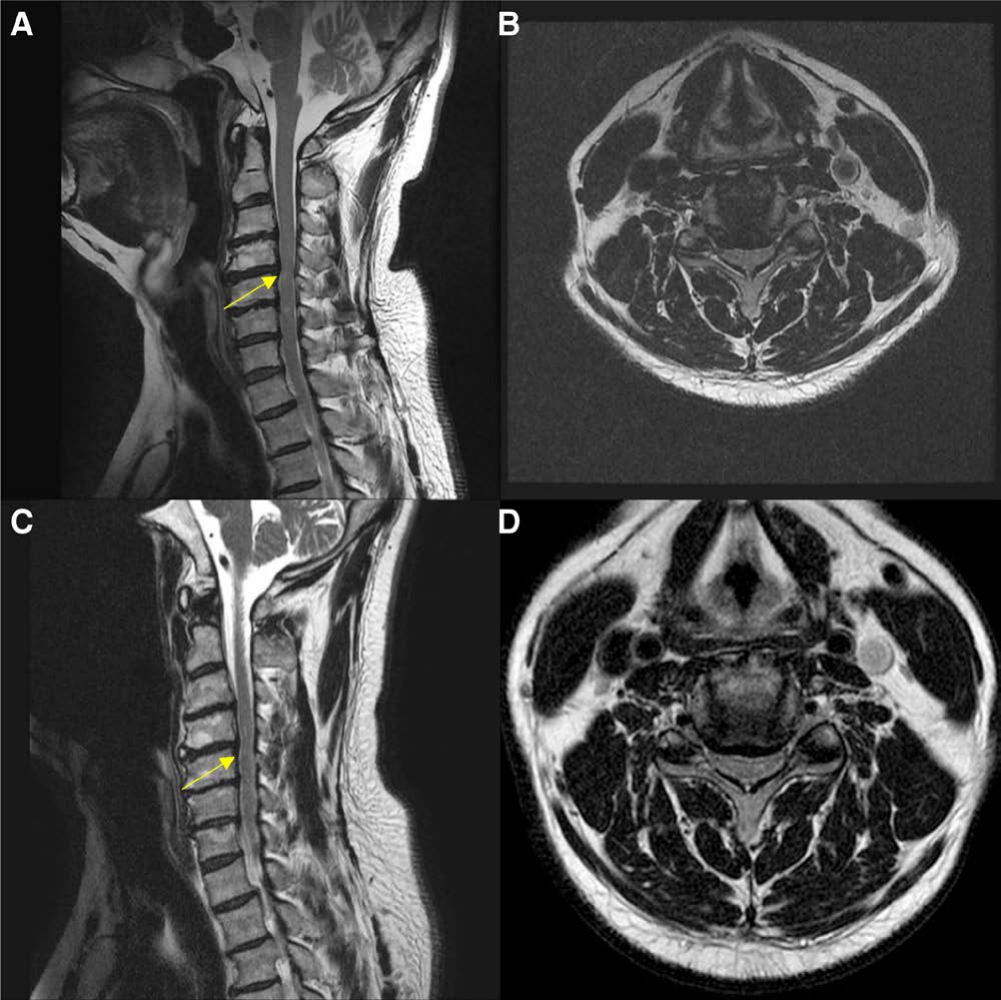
Case 2: (A) C-SPINE MRI. (B) Scanned on March 21, 2023. (C and D) Scanned on October 06, 2023. MRI = magnetic resonance imaging.
